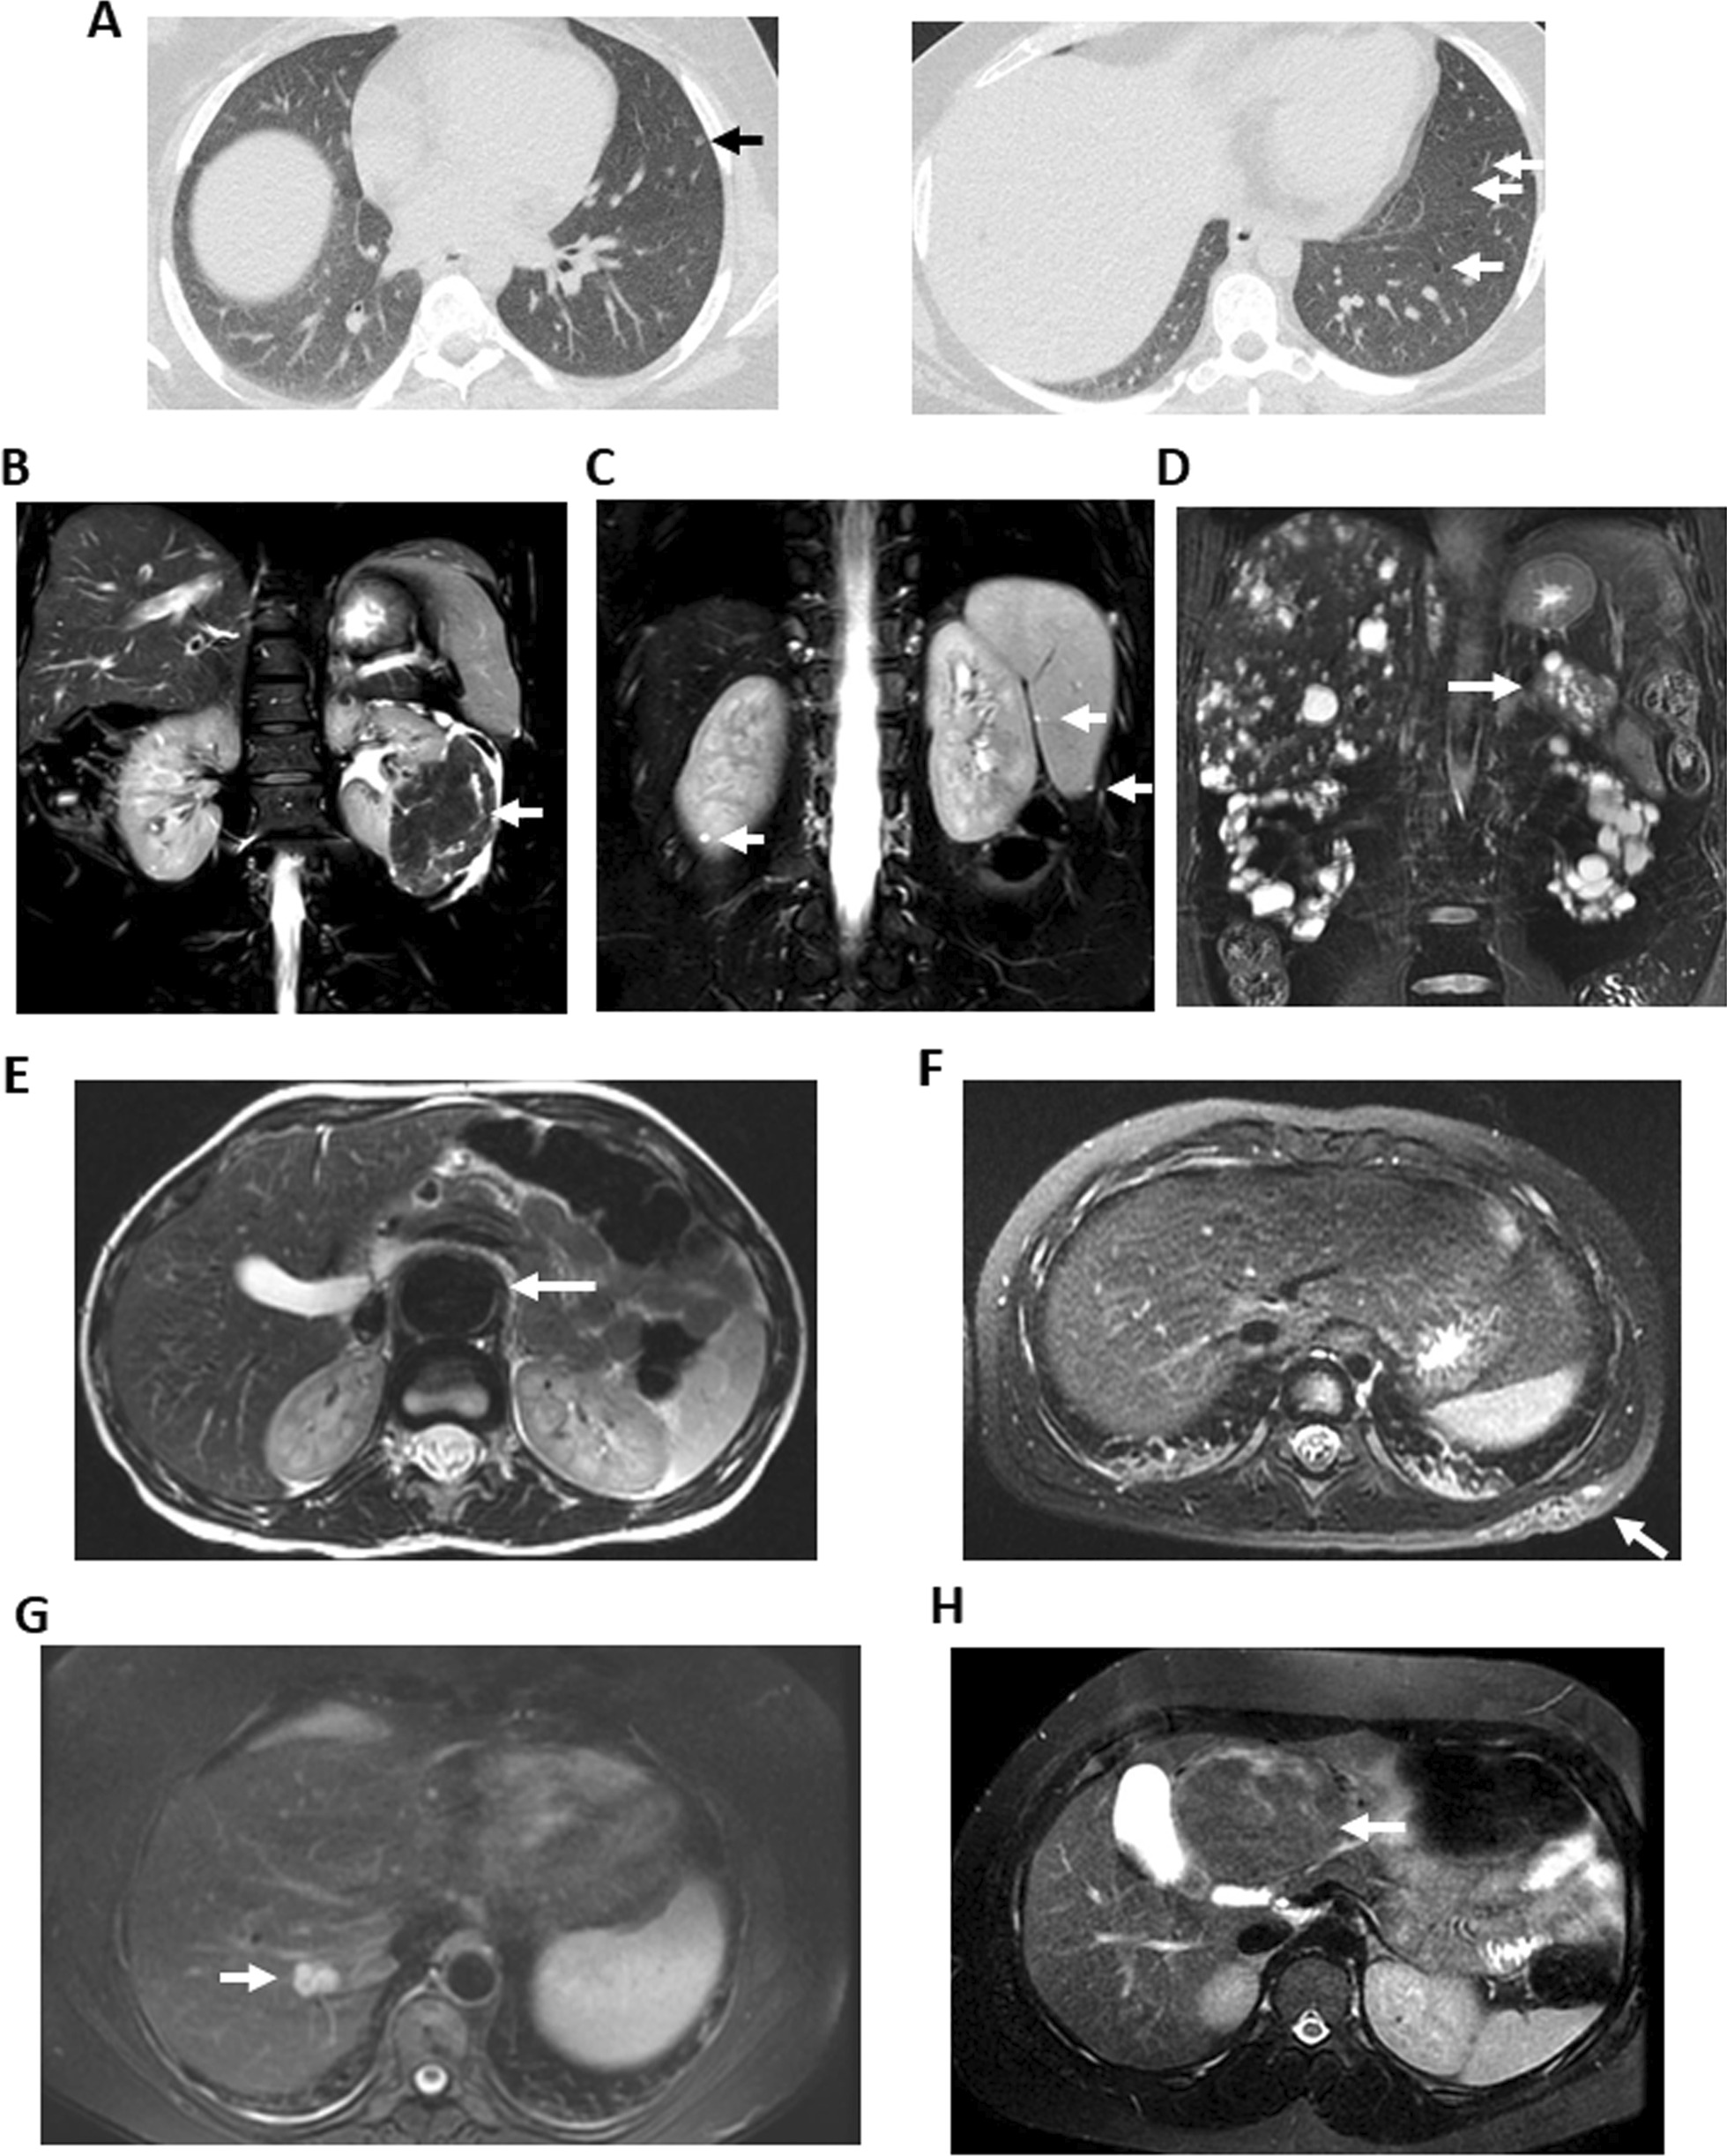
Representative imaging findings in TSC. A Axial CT chest in lung windows of 34 year old female with pulmonary nodules (black arrow) and cysts (white arrows). B 29 year old female with large AML arising from left kidney (arrow) on coronal T2-weighted fat saturated MRI. C 7 year old male with simple splenic and renal cysts (arrows) on axial T2-weighted fat saturated MRI. D 42 year old male with innumerable renal, hepatic, and pancreatic (arrow) cysts on coronal T2-weighted fat saturated MRI. Patient has had no genetic testing but has a clinically-suspected diagnosis of TSC2-PKD contiguous gene syndrome. E 5 year old male with abdominal aneurysm (arrow) on axial T2-weighted abdominal MRI. F 5 year old female with lymphatic malformation (arrow) on axial T2-weighted fat saturated MRI. G 53 year old female with hepatic hemangioma (venous malformation, arrow) on axial T2-weighted fat saturated abdominal MRI. H 15 year old female with hepatic adenoma (arrow) on axial T2-weighted fat saturated abdominal MRI
